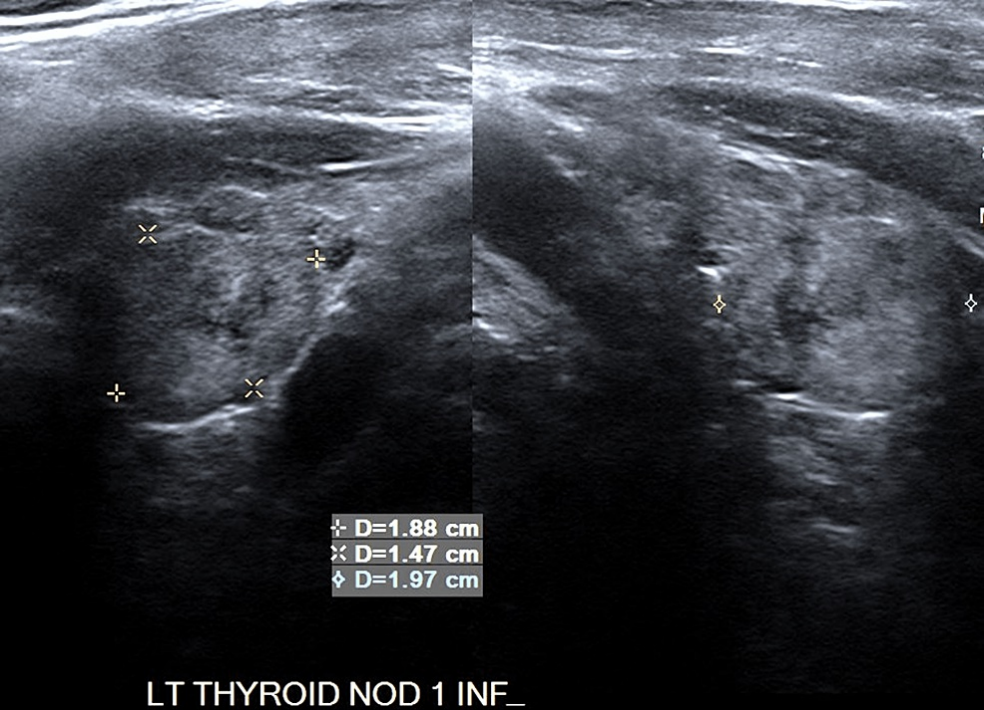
Multinodular Left (Inferior) Thyroid Ultrasound Lesions are marked (x) on image.
